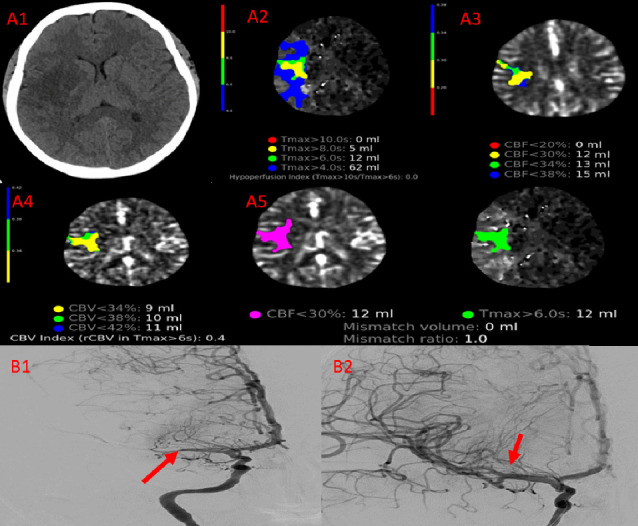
A 29-year-old female with rheumatic heart disease and chronic atrial fibrillation developed facial drooping and acute hemiparesis 24 h after redo mitral valve replacement. Computed tomography of the brain and angiography revealed acute large right middle cerebral artery (MCA) territory ischemic insult, large vessel occlusion involving the M1 segment of the right MCA, and impaired perfusion parameters (A1–5). Cerebral angiography showed total occlusion of M1 branch of the right MCA (B1). Image B2 shows the thrombolysis in cerebral infarction Flow III after thrombus aspiration, with areas of narrowing consistent with nonflow limiting vasospasm. The patient was discharged without any residual weakness.
